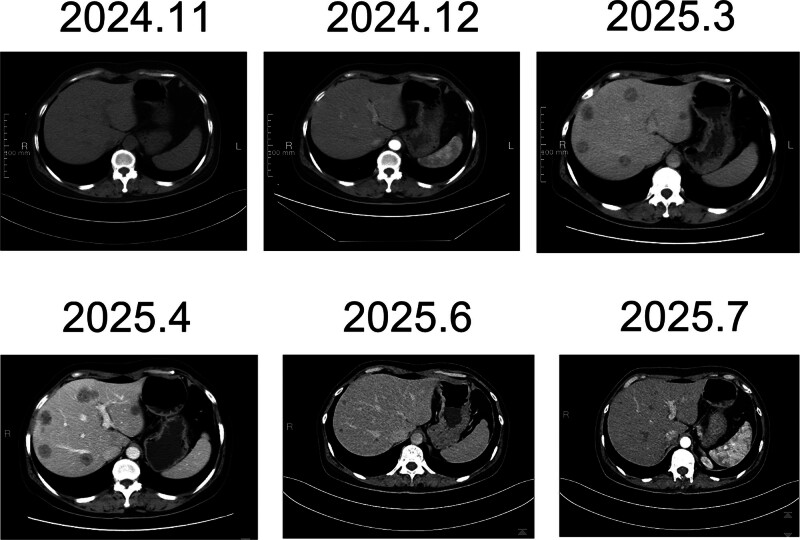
CT diagnoses of the patient during treatments. CT = computed tomography.
